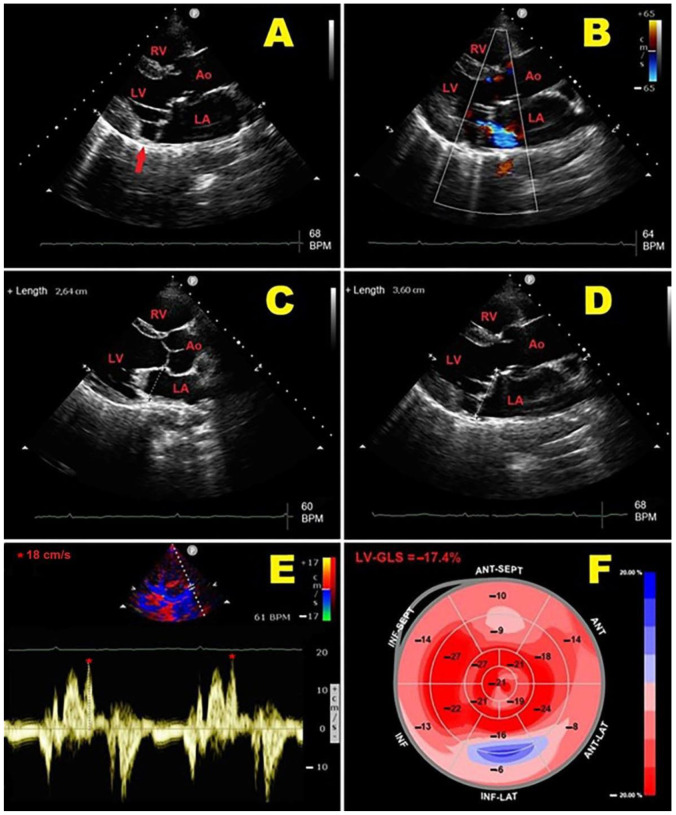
Representative example of main echocardiographic findings detectable in MVP individual with MAD. (A): Transthoracic parasternal long-axis view showing MAD (red arrow) and bileaflet MVP. (B): Transthoracic parasternal long-axis view showing bileaflet MVP with mild-to-moderate mitral regurgitation. (C): End-diastolic MVA diameter measurement from transthoracic parasternal long-axis view. (D): End-systolic MVA diameter measurement from transthoracic parasternal long-axis view, with evidence of paradoxical systolic MVA expansion. (E): PW-TDI of lateral mitral annulus, demonstrating high-velocity (>16 cm/s) mid-systolic spike in tissue Doppler velocity profile of mitral valve annulus (Pickelhaube sign). (F): LV-GLS bull’s eye plot assessed by 2D-STE analysis. In present case, mild impairment of LV-GLS (absolute value = −17.4%) was primarily related to LV-BLS attenuation, with peculiar involvement of LV-basal inferolateral wall. 2D, two-dimensional; Ao, aorta; BLS; basal longitudinal strain; GLS, global longitudinal strain; LA; left atrium; LV, left ventricle; MAD, mitral annular disjunction; MVA, mitral valve annulus; MVP, mitral valve prolapse; PW, pulsed-wave; RV, right ventricle; STE, speckle tracking echocardiography; TDI, tissue Doppler imaging. The symbol * indicates the mid-systolic peak velocity in tissue Doppler velocity profile of mitral valve annulus.
